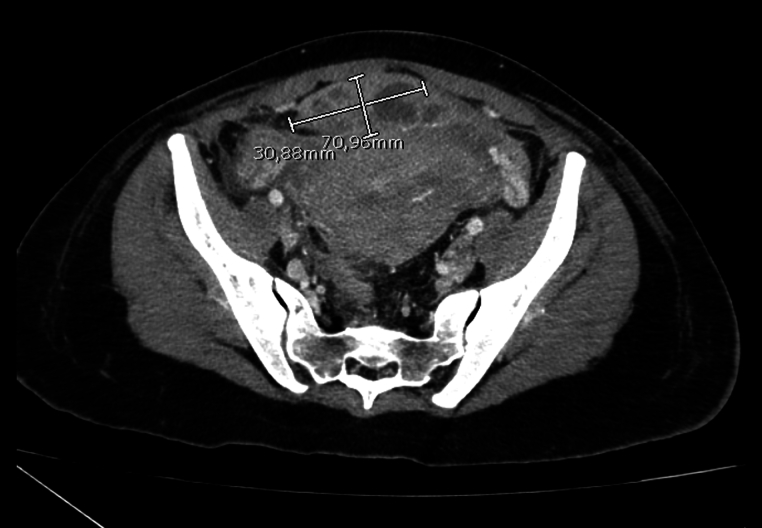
Coronal view of the CT abdomen and pelvis with IV contrast performed demonstrating an oval alteration within the medical umbilical ligament anterior to the uterus and superior to the bladder, measuring approximately 30 × 70 mm in axial diameters and characterized by uneven contrastographic impregnation with hypodense areas.
